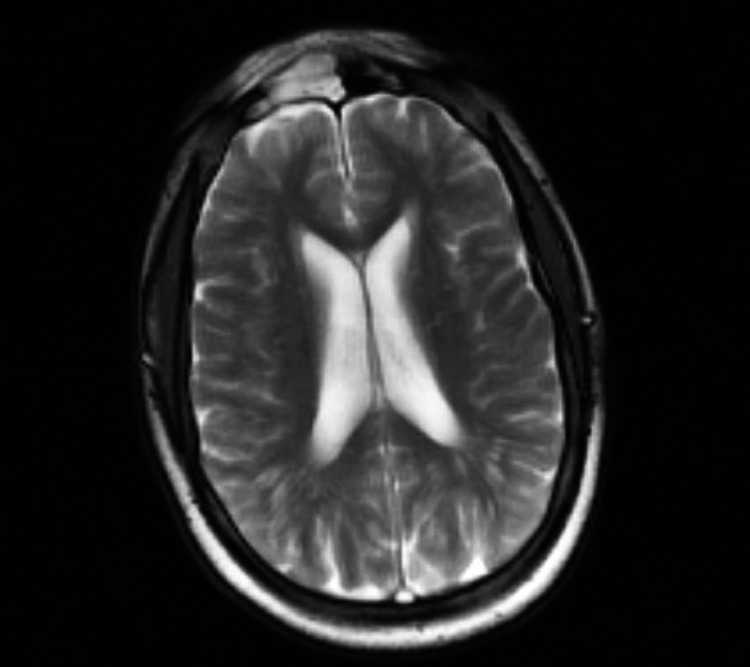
MRI of the brain showing diffuse meningeal enhancement
